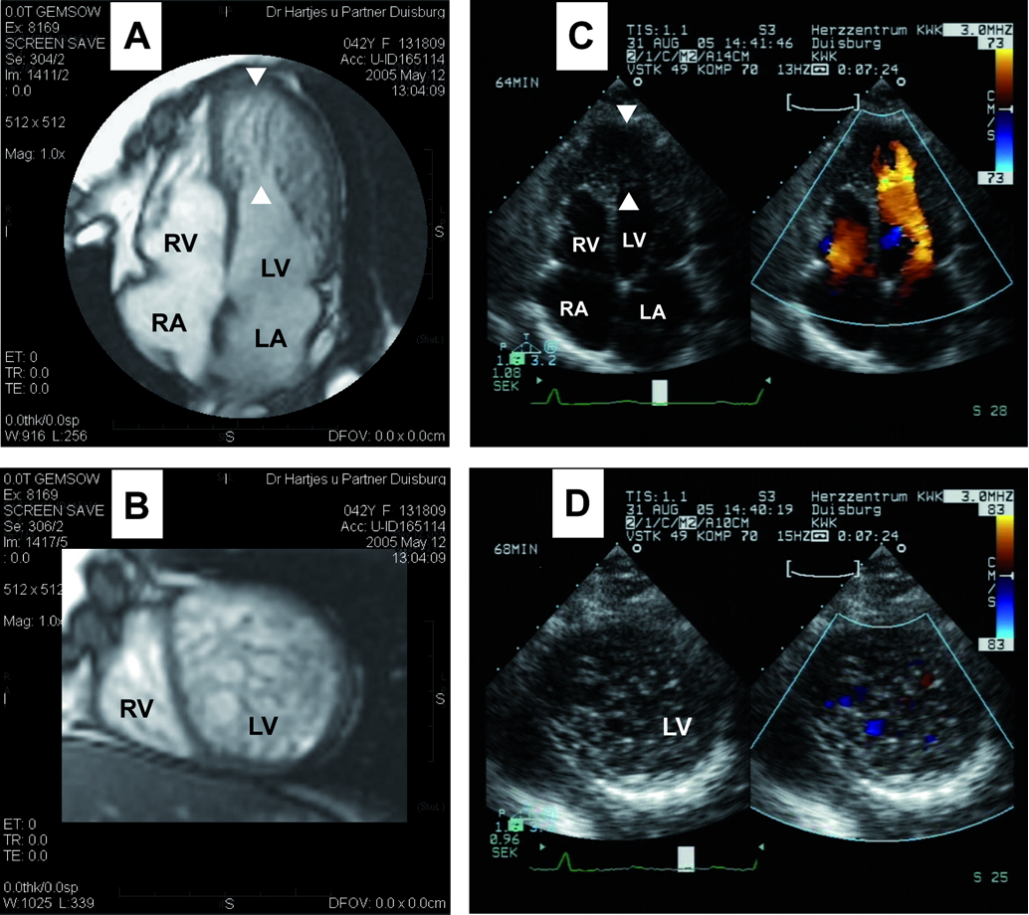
Imaging of NVM in patient III:8 of family DU-11. A, Magnetic resonance image: long-axis plane in diastole. Prominent myocardial trabeculations and deep intertrabecular recesses are seen in the apical half of the right and left ventricle as indicated by arrows. B, Magnetic resonance image: short-axis plane in diastole. In the apex region the cavum and the myocardial wall can not clearly be distinguished. Extensive trabeculations in this region produce a sponge-like appearance of the myocardium. C, Two-dimensional echocardiograph: apical four-chamber view in diastole with (right) and without (left) color flow imaging. A massive apical thickening is seen without any evidence of trabeculations in the left image. In contrast, color flow imaging (right image) shows more clearly the recesses extending deeply into the myocardial wall. D, Two-dimensional echocardiograph: parasternal short-axis view in diastole with (right) and without (left) color flow imaging. The left image shows a mesh-like morphology in the mid-region of the left ventricle. The cavum is not clearly discernable. The right image demonstrates numerous small blood-flow eddies within the sponge-like myocardium (as shown by color flow imaging). LV, left ventricle; RV, right ventricle; LA, left atrium; RA, right atrium.
